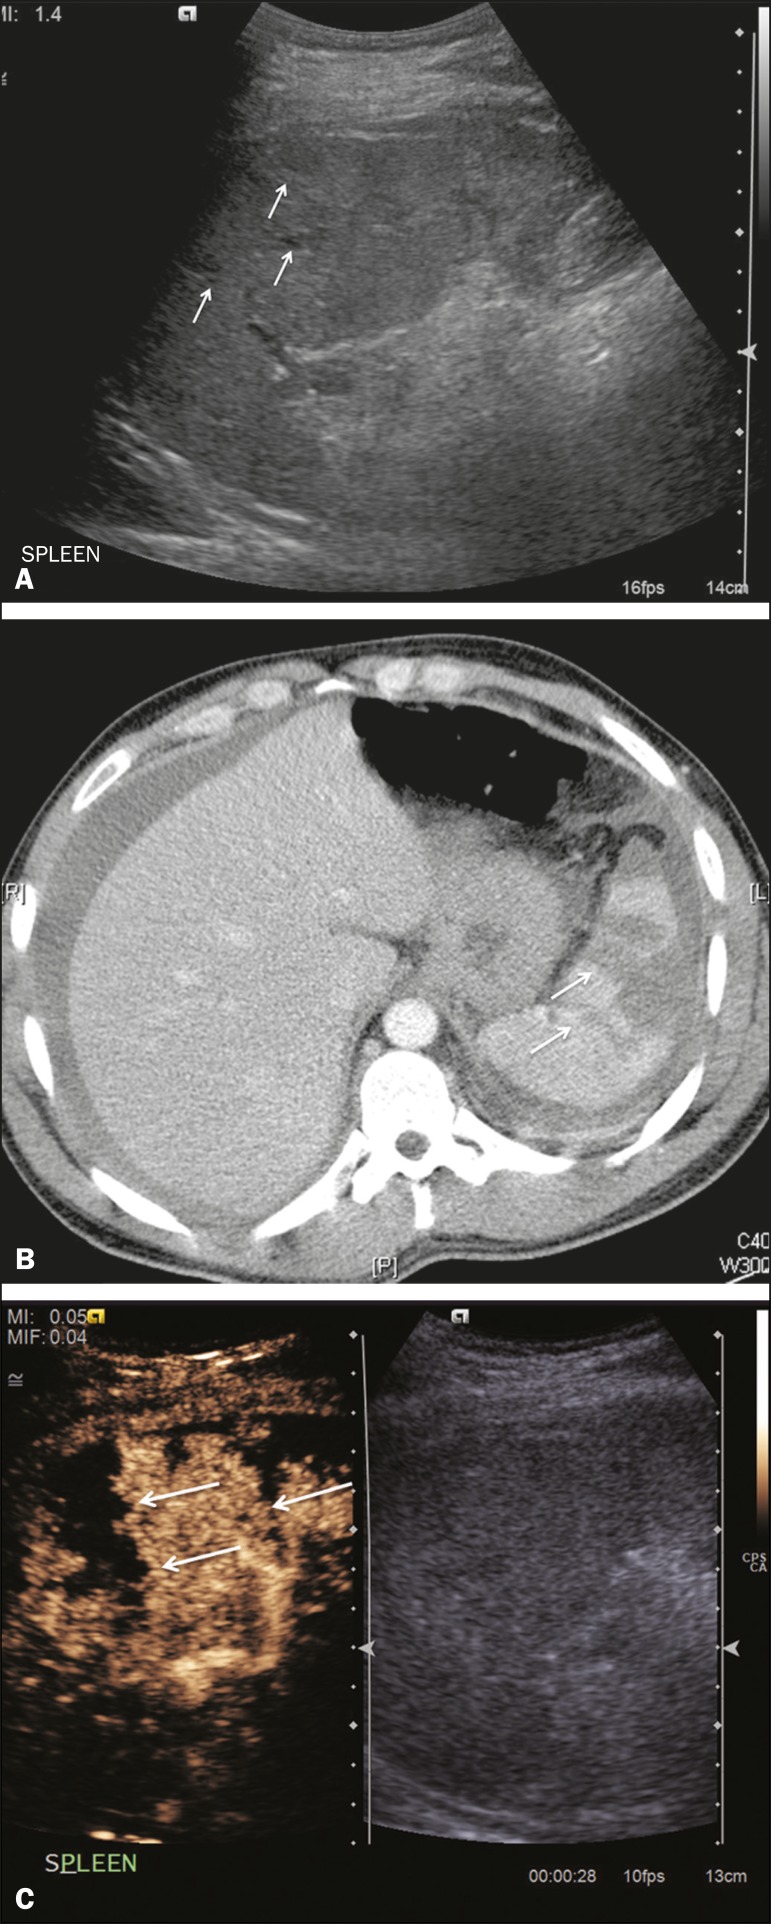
Splenic trauma. A: B-mode ultrasound showing subtle
